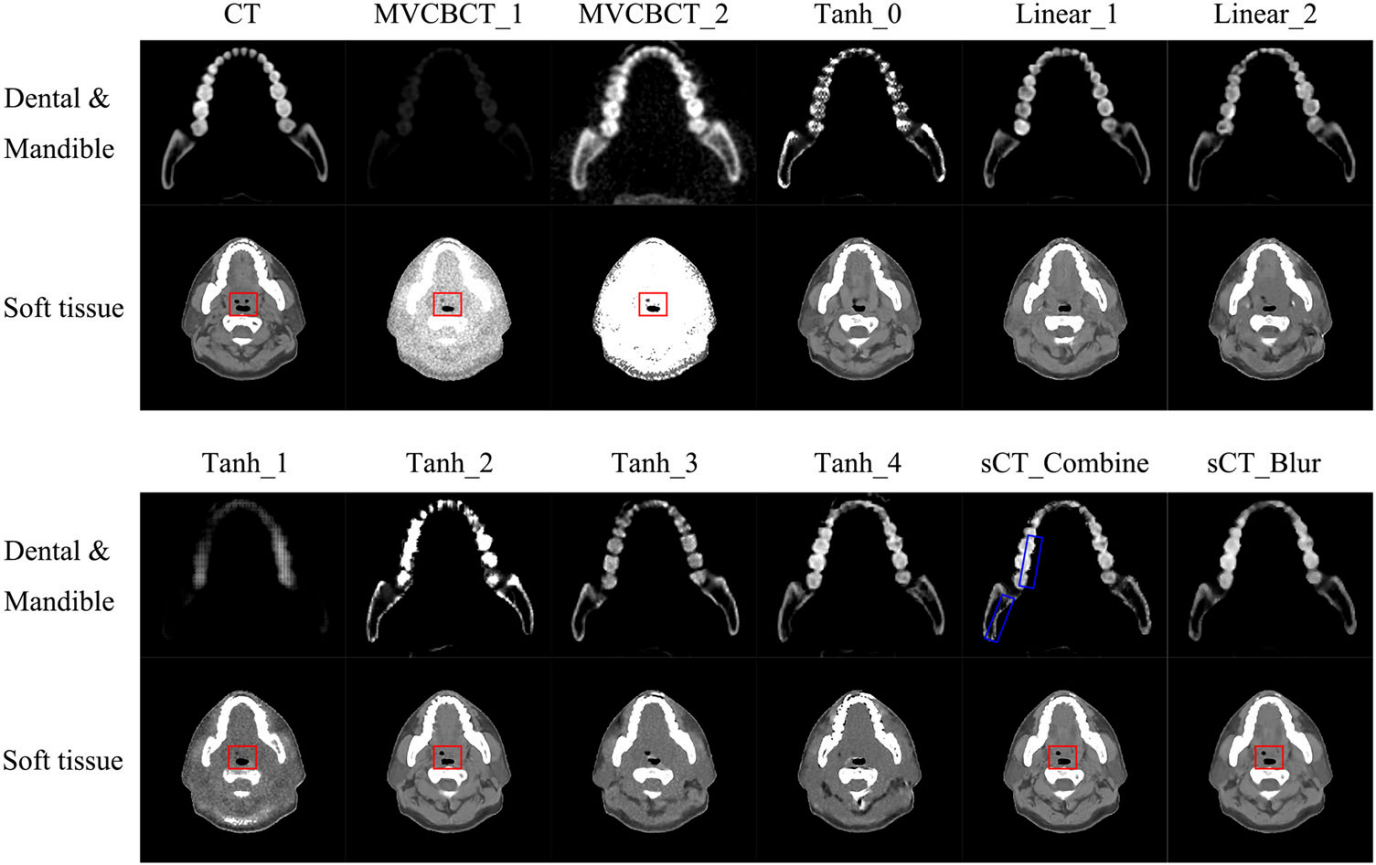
Comparison of the CT, MVCBCT, and sCT images. The display windows are [−260, 340] HU for soft tissue and [500, 2500] HU for dental tissue and the mandible. Different sCT images have their own advantages and disadvantages in various tissue regions, while both soft and bony tissues in sCT_Blur are close to the planning CT images. CT, computed tomography; MVCBCT, megavolt cone‐beam CT; sCT, synthetic computed tomography.
